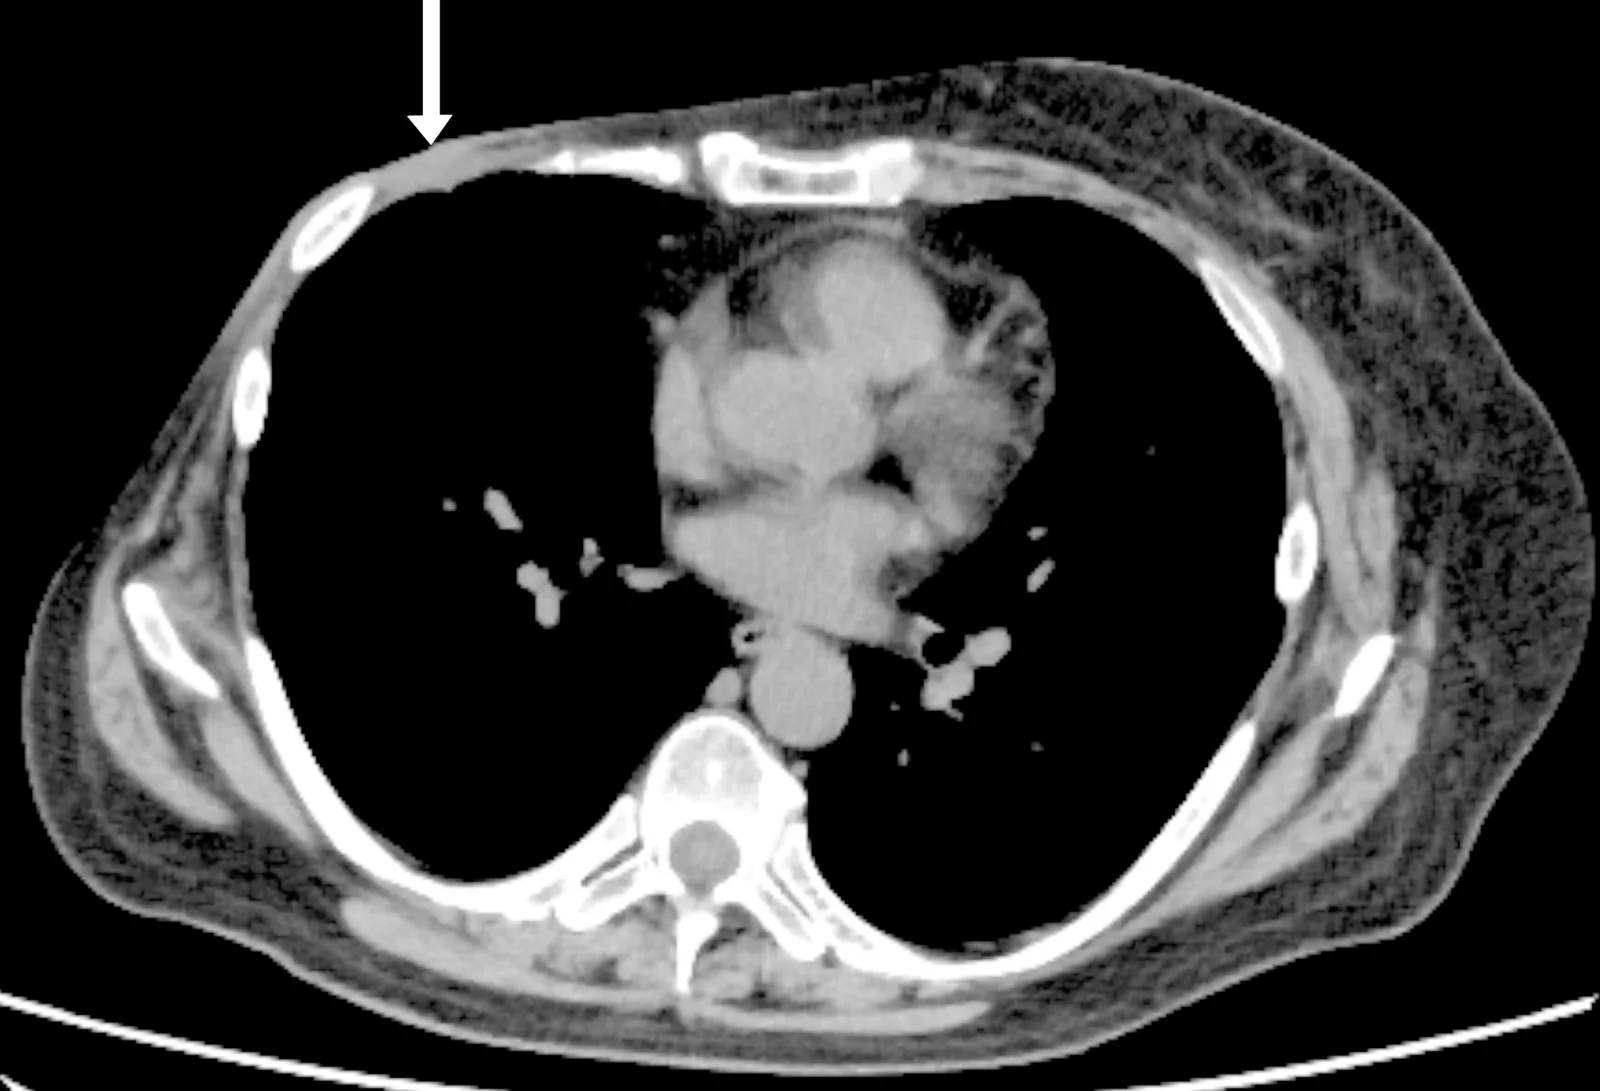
Axial chest CT showing soft-tissue change in the skin and subcutaneous tissue around the right sixth rib corresponding to the clinically observed ulcer, without an obvious mass suggestive of chest-wall recurrence
